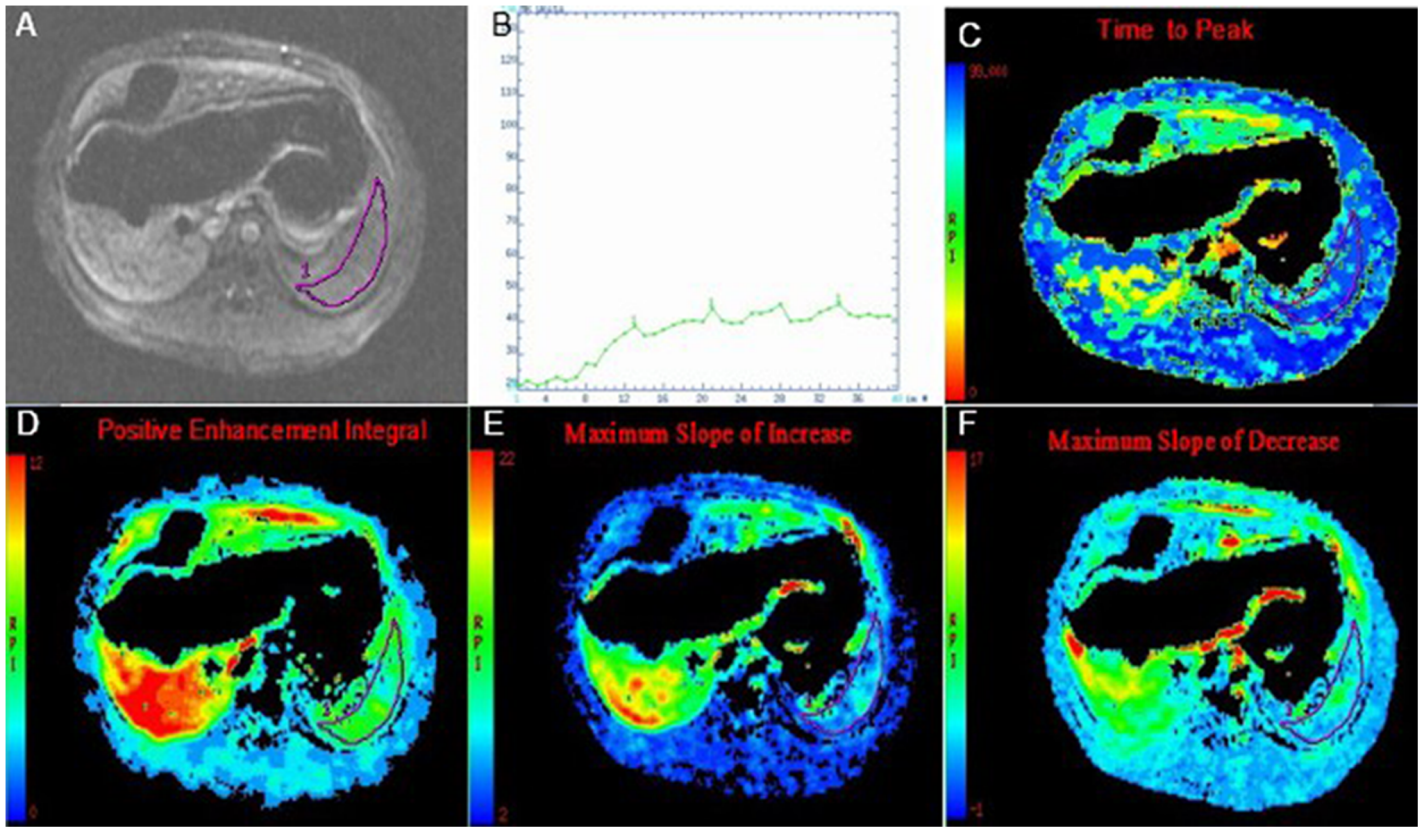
An example of spleen DCE-MRI data measurement. The image (A) shows that region of interest (ROI) has been carefully drawn on dynamic contrast-enhanced magnetic resonance image (DCE-MRI), and the obtained signal-time intensity curves (STC, B) of spleen parenchyma are derived from the standard software package. Graphs show the color maps of time to peak (C), positive enhancement integral (D), and maximum slope of increase (E) and decrease (F).
